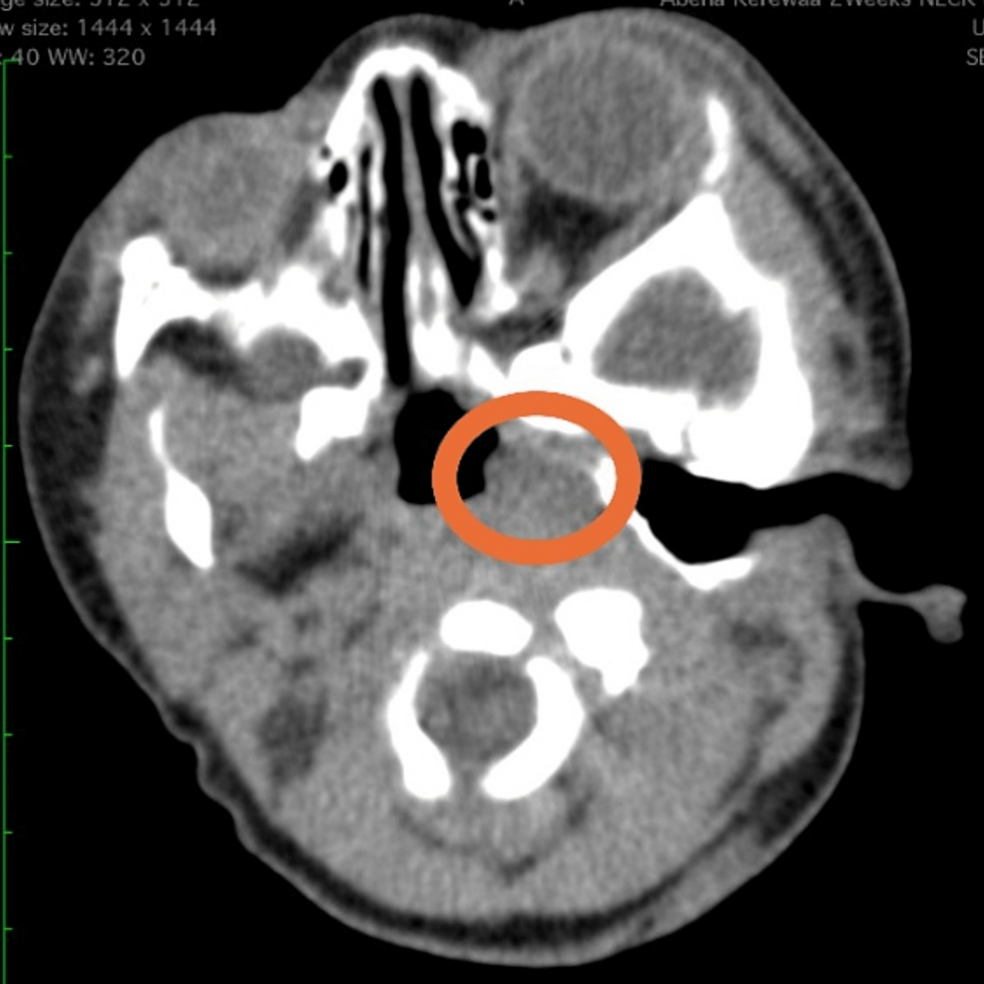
Axial CT scan of the paranasal sinuses and pharynx showing a mass in the left half of the nasopharynx (encircled) CT: computed tomography
